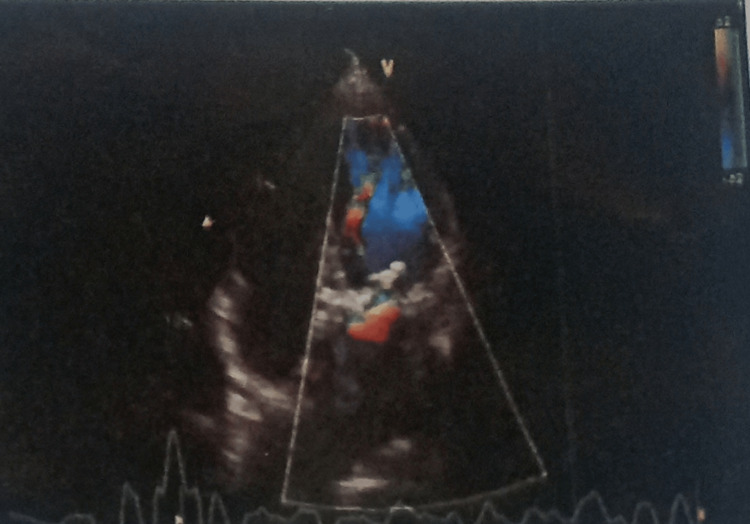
Color Doppler across the mitral valve in the patient with mitral valve stenosis
